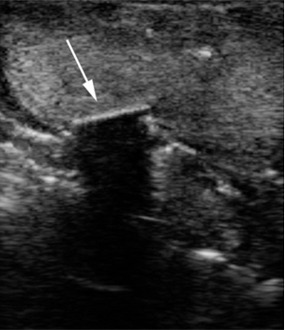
Arrow points to a wooden foreign body on the ultrasound image.
